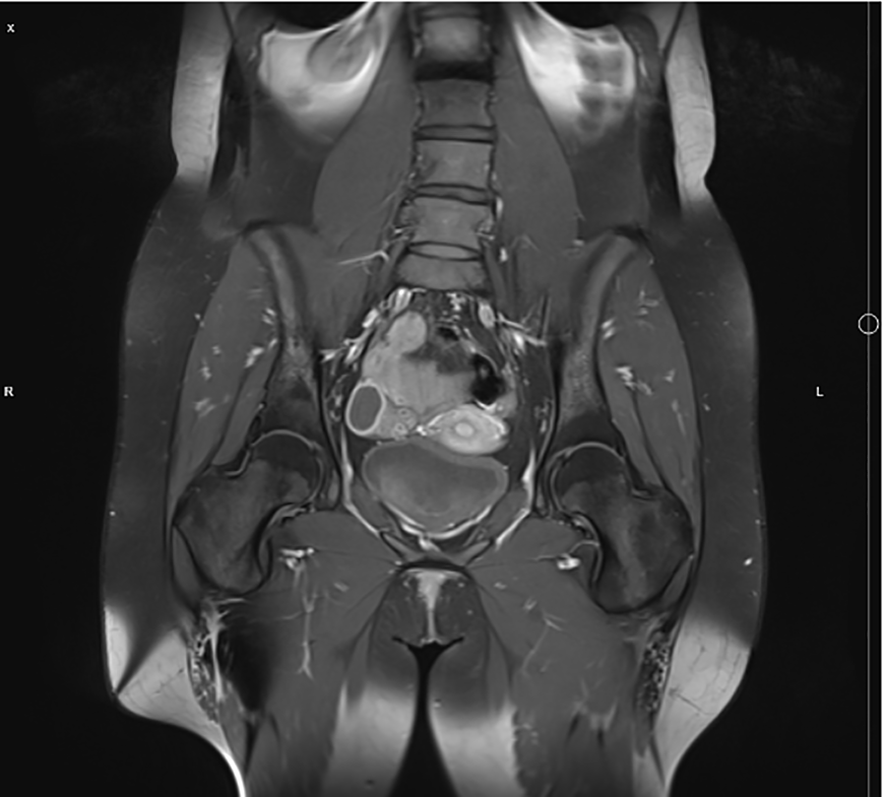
Pelvic MRI showing signs of bone marrow edema in both femoral heads.
